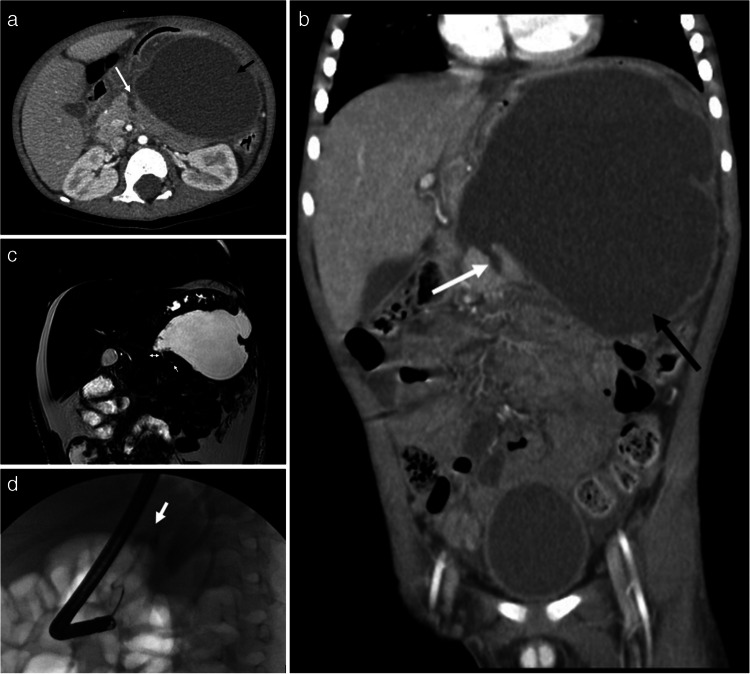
A 3-year-old male with a history of developmental delay presented with a new onset of repeated vomiting after feeds and on further evaluation was found to be in an abusive home environment. CT of the abdomen and pelvis with IV contrast in portal venous phase was obtained. a Axial image shows a deep laceration through the pancreas (*white arrow*) and a pancreatic pseudocyst anterior to the pancreatic tail (*black arrow*) and posterior to the stomach, which is partially compressed and anteriorly displaced by the pancreatic pseudocyst. b Coronal image shows a deep laceration through the pancreas (*white arrow*) which appears to communicate with a pancreatic pseudocyst (*black arrow*). c Coronal 3D thin slice MRCP image shows partial visualization of the main pancreatic duct in the pancreatic tail (*white arrow*) and a pancreatic laceration which communicates with the pancreatic pseudocyst near the main pancreatic duct course (*white double arrow*) concerning for a ductal tear. d Coned image from an ERCP with contrast injection into the main pancreatic duct shows a focal amorphous region of contrast extravasation (*black arrow*) in the region of the superiorly positioned pseudocyst confirming a tear of the main pancreatic duct which communicates with the pseudocyst
